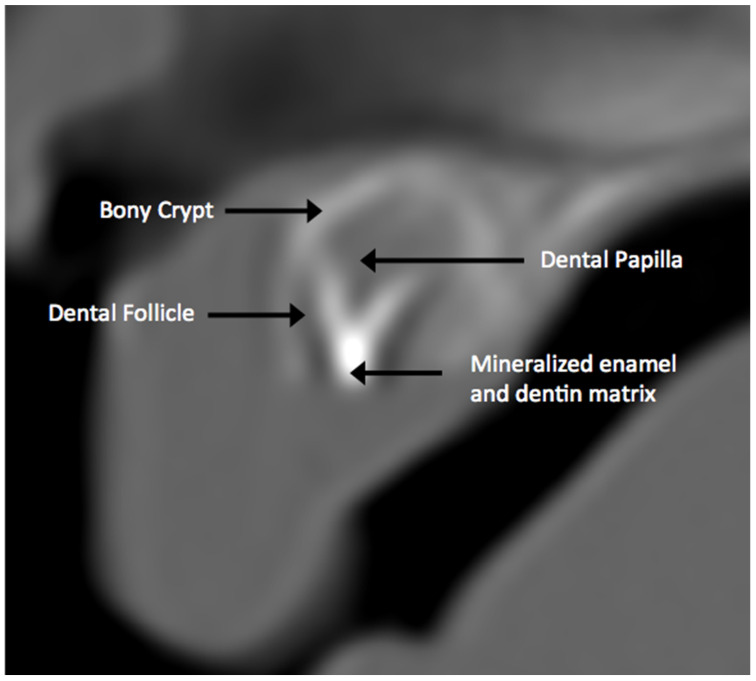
Post-appositional tooth germ anatomy of a central incisor on CT. Note the hyperdense appearance of the mineralized bony crypt and mineralizing crown (enamel and dentin) of the tooth relative to the hypodense appearance of the dental follicle and papilla.
